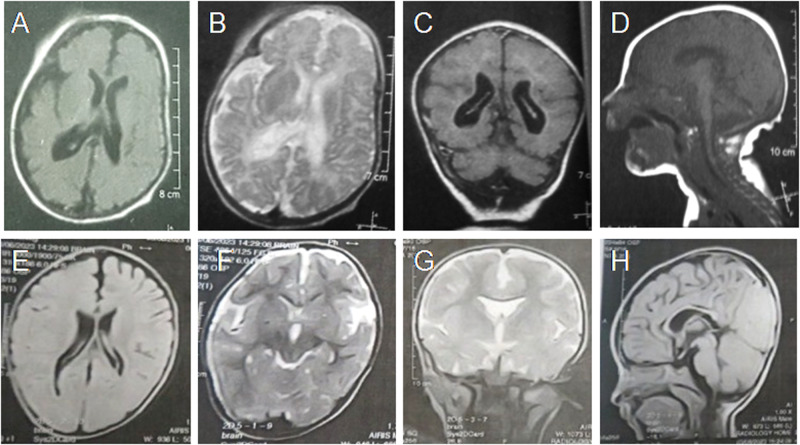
Brain MRI findings of our patients. First row is for Patient 1 at the age of 3 months showing A, B mild asymmetric ventriculomegaly, under-opercularization, C, D hypogenesis of corpus callosum and cerebellar and brainstem atrophy. Second row is for Patient 5 at the age of 15 months that showed E, F asymmetric ventriculomegaly, under-opercularization, G cavum septum pellicidum, and H hypogenesis of corpus callosum
